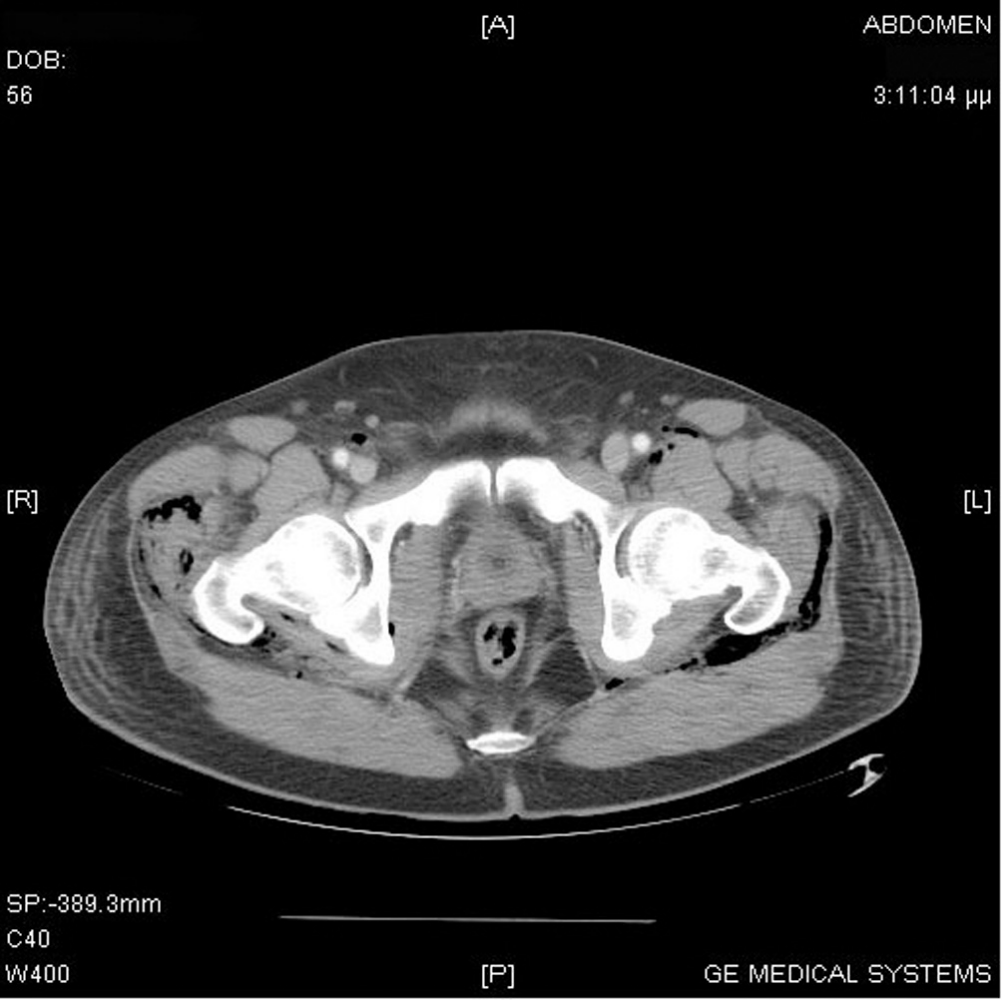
CT of the pelvis and lower extremities demonstrating extensive air and fluid through the upper part of the thighs. Air and fluid was also depicted along the fascias of the muscles on both buttocks.
